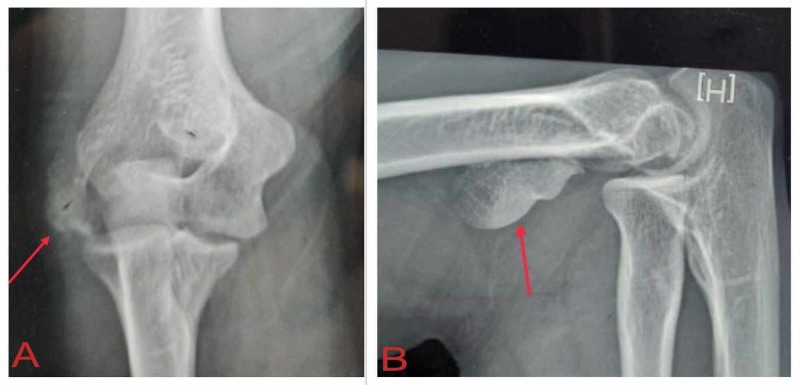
Preoperative X-rays of the right elbow. The arrows show the fractured capitellar fragment in the (A) anteroposterior and (B) lateral views.
